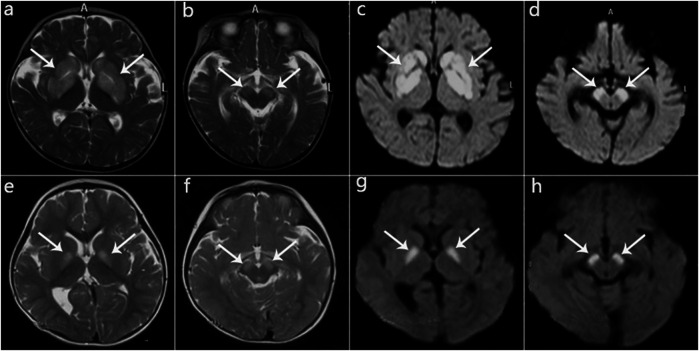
MRI manifestations of 2 patients (Patient 2, Patient 5) during disease onset. (a‐d): Patient 2 at age 10 months; hyperintensity of the globus pallidus, putamen, caudate nucleus, and cerebral peduncle on T2WI (a,b) and DWI (c,d). (e‐h): Patient 5 at age 13 months; hyperintensity on T2WI (e,f) and DWI (g,h) in the globus pallidus and cerebral peduncle.
